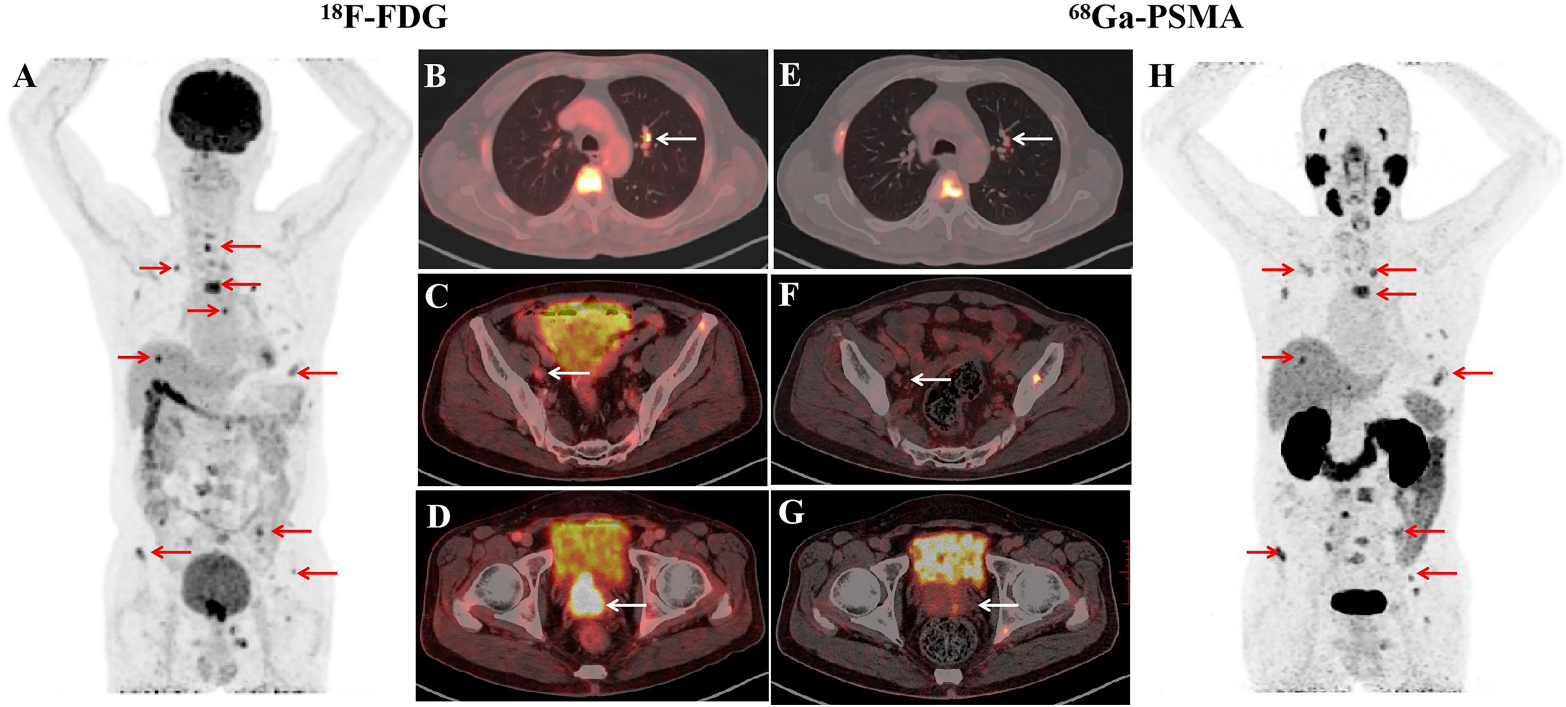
Imaging studies before the treatment of ADT combination with PARPi. In PET/CT images, PET maximum-intensity-projection image (MIP) (A), Fused axial (B–D) showed intense diffuse accumulation of FDG in prostate mass (D, white arrow), lymph nodes (C, white arrow), pulmonary nodules (B, white arrow), bone lesions (A, red arrows). PSMA PET/CT images showed abnormal uptake of in bone lesions (H, red arrows), slight development of prostate mass (G, white arrow), lymph nodes (F, white arrow), pulmonary nodules (E, white arrow).
